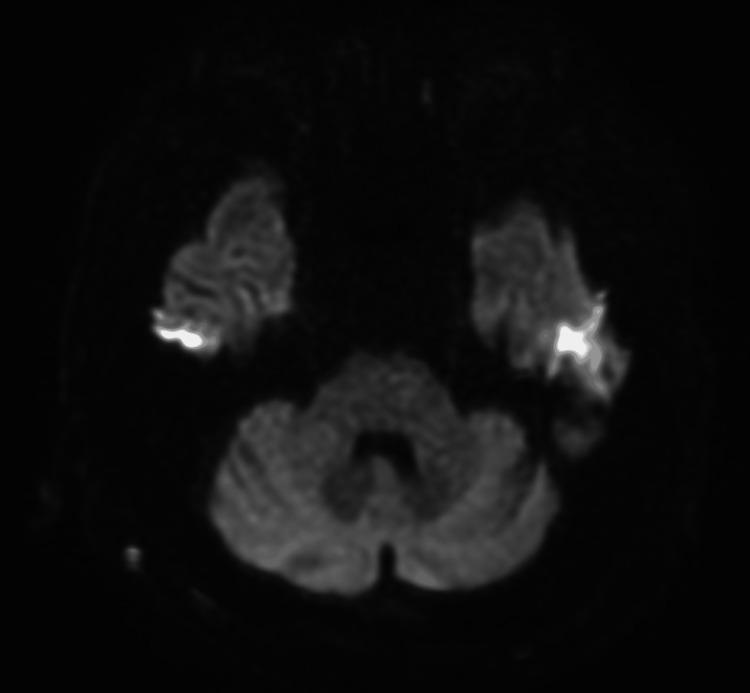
Brain MRI (DWI of the brain) DWI of the brain reveals no acute stroke findings in the medulla or cerebellum. MRI: magnetic resonance imaging, DWI: diffusion-weighted imaging
